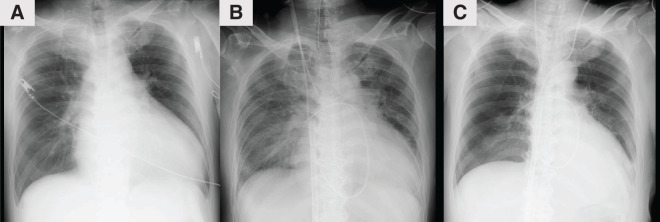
Change over time in chest X-ray. Serial chest X-rays showing changes over time: (A) at admission, (B) prior to LVAD implantation, and (C) 5 days after LVAD implantation. LVAD, left ventricular assist device
